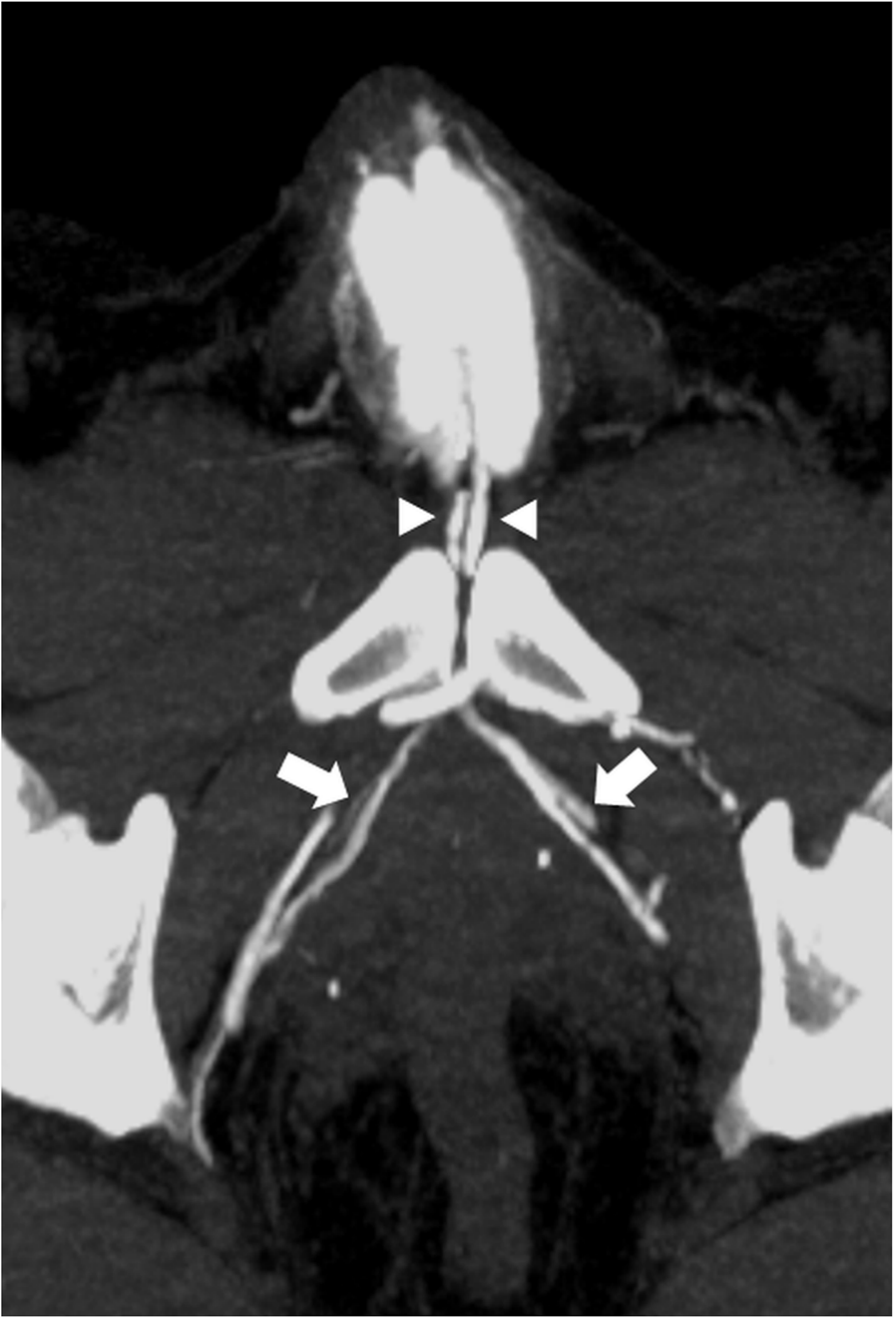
Contrast enhanced computed tomography cavernosography (maximum intensity projection) demonstrating major venous leakage from deep dorsal penile veins via bilateral periprostatic veins (arrows) draining into internal pudendal veins and bilateral iliohypogastric veins. Of interest, paired deep dorsal penile veins were found (arrowheads)
